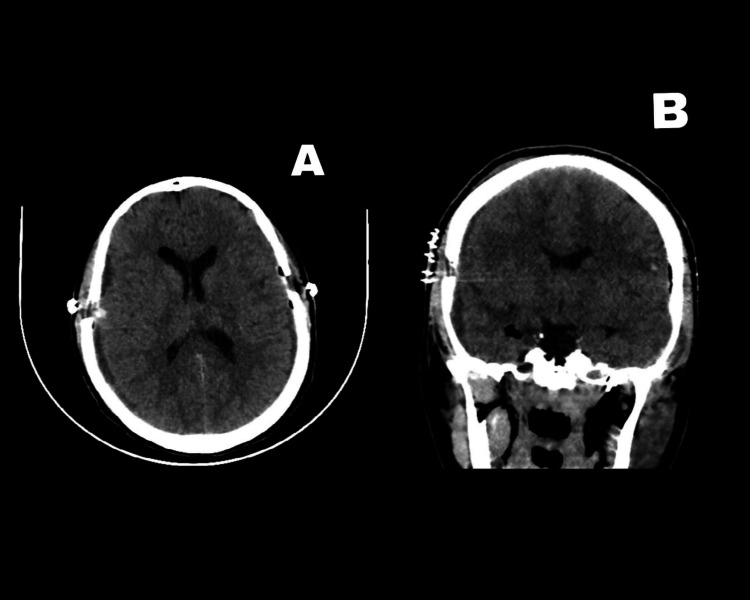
Postoperative head CT scan showing the resolution of the subdural hemorrhage Figure 2A shows the axial view. Figure 2B shows the coronal view CT: computed tomography
